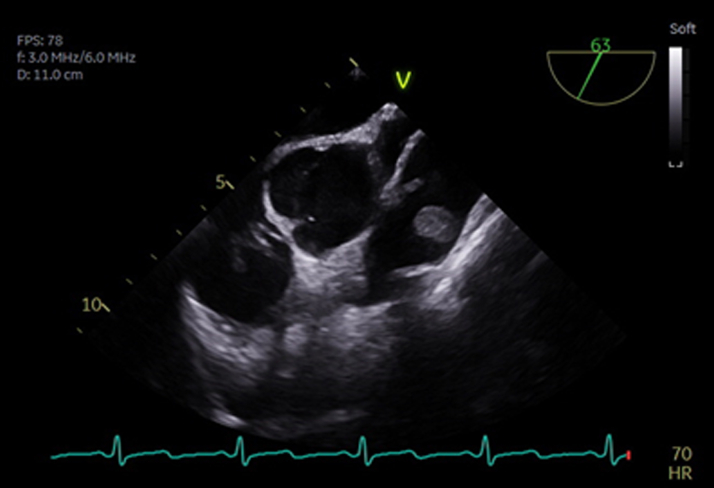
Intraoperative Transesophageal Echocardiography Images: Right-Sided Papillary Fibroelastoma
